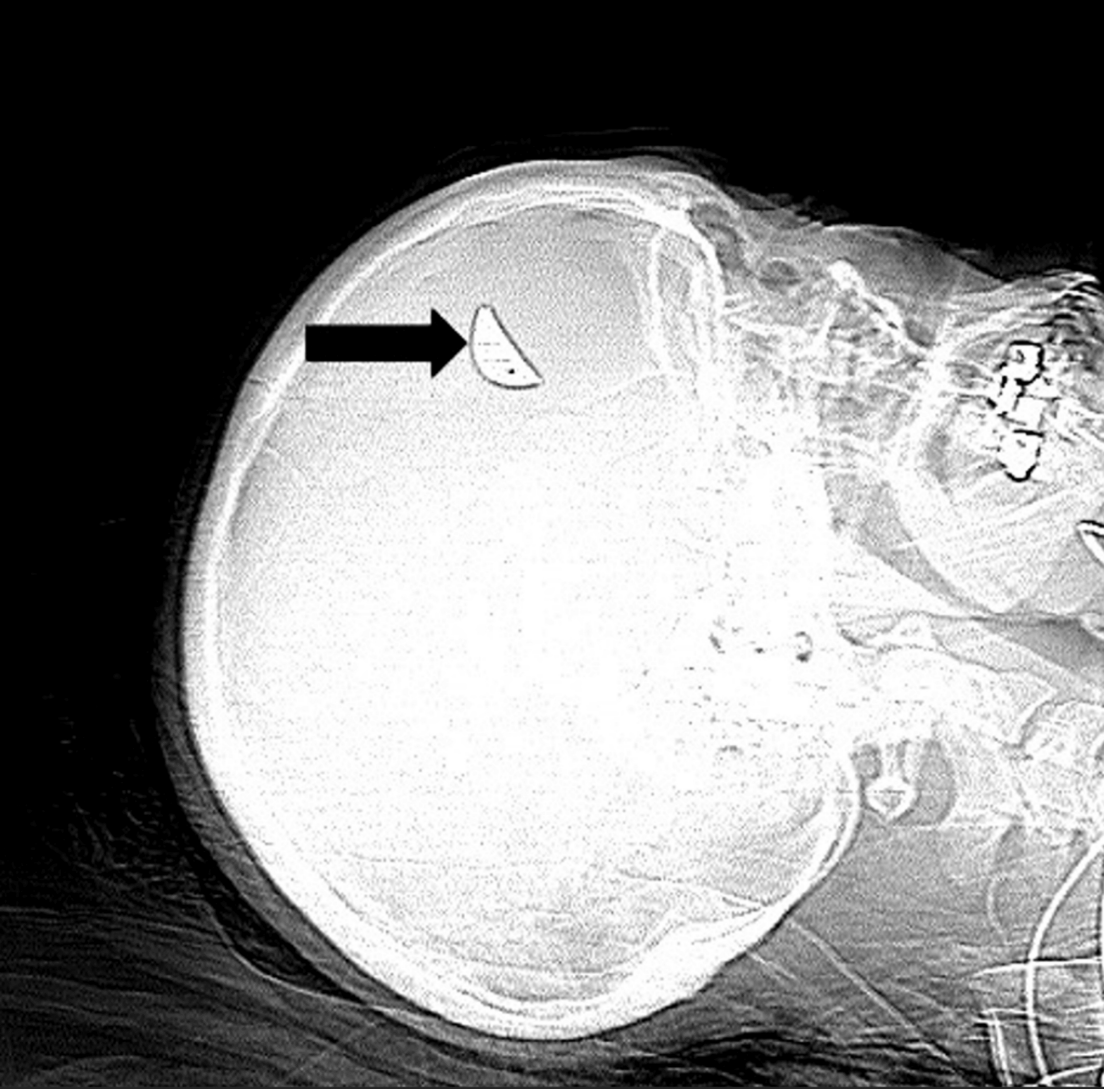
Scout image of the intracranial foreign body preoperatively Preoperative scout X-ray image demonstrating a dense, crescent-shaped intracranial foreign body measuring approximately 2 cm, located in the dorsolateral aspect of the right frontal lobe
